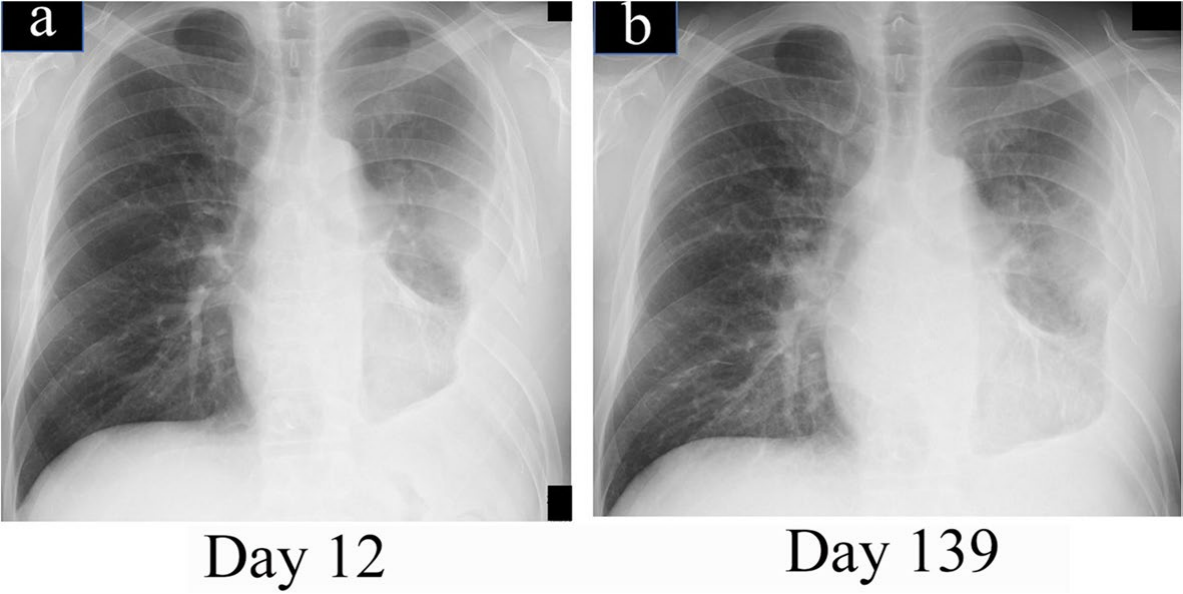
Changes in chest X-ray after closure surgery. Chest X-ray at discharge (12 days after closure surgery). b 4 months after discharge (139 days after closure surgery). Improvement in pulmonary expansion was observed
